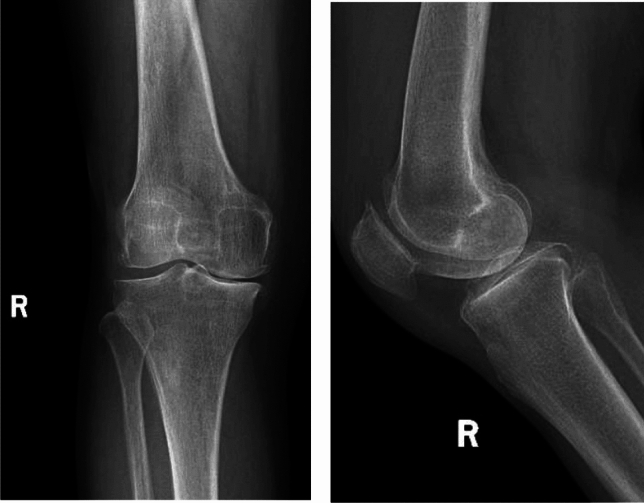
Anterior and lateral radiography films of the knee joint before operation showing the changes in osteoarthritis of the right knee joint, especially in the medial compartment
